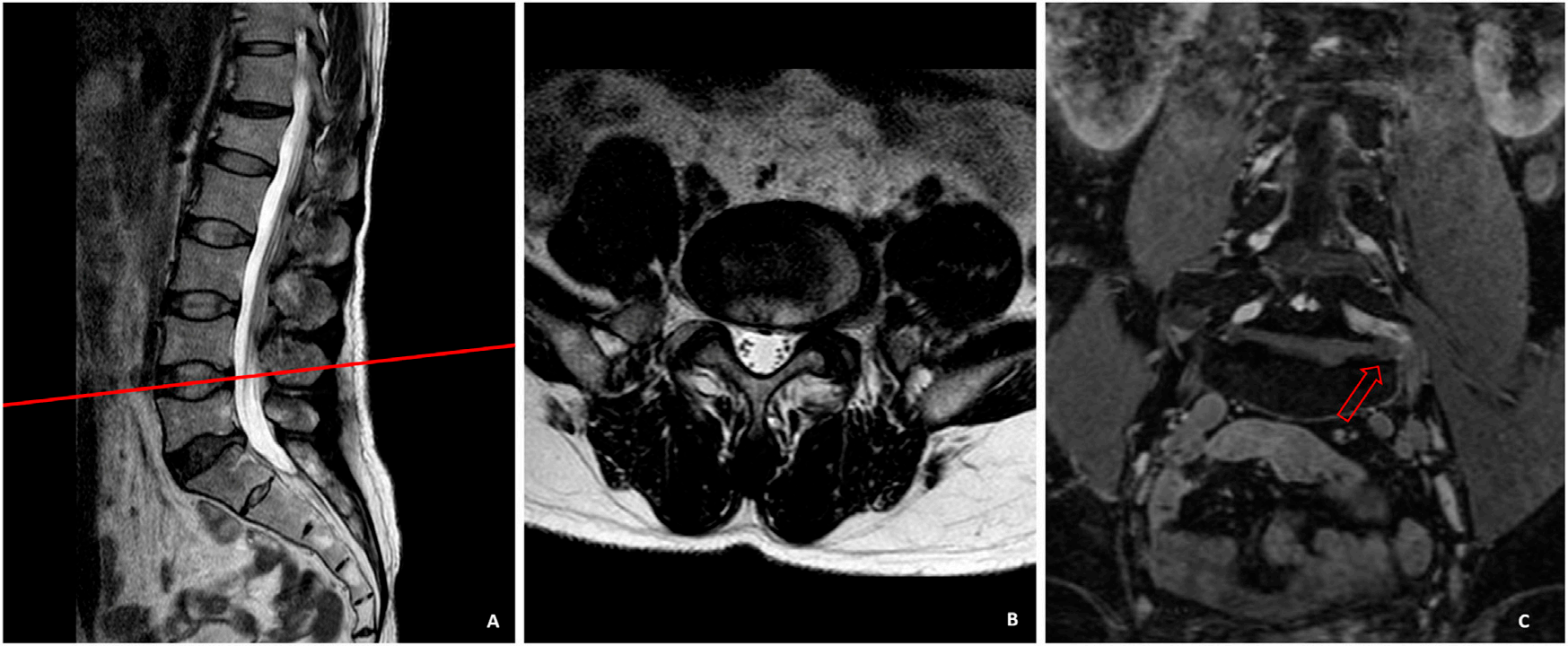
In sagittal (A) and cross-sectional (B) MRI, the herniated nucleus pulposus tissues near the intervertebral foramen were easily ignored. The compression of the nucleus pulposus of L4/L5 segment to the left L4 nerve root outside the intervertebral foramen could be intuitively observed on the 3D-DESS image (C).
